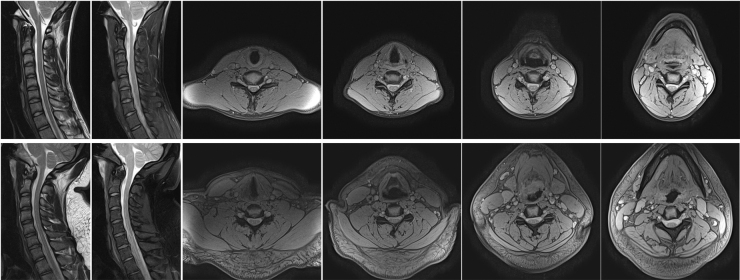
Sample c-spine images acquired using various sequences from a player without (top row) and with (bottom row) concussion history. The presented images from left to right are: T2w in sagittal orientation; fat suppressed T2w in sagittal orientation; and multi-echo T2*w in axial orientation at four different locations. The assigned grades in the player without concussion history were II, III, II, III, II, and I at C2/3, C3/4, C4/5, C5/6, C6/7, and C7/T1, respectively; the assigned grades in the player with concussion history were I, III, III, III, III, and I at C2/3, C3/4, C4/5, C5/6, C6/7, and C7/T1, respectively. T2w, T2-weighted.
